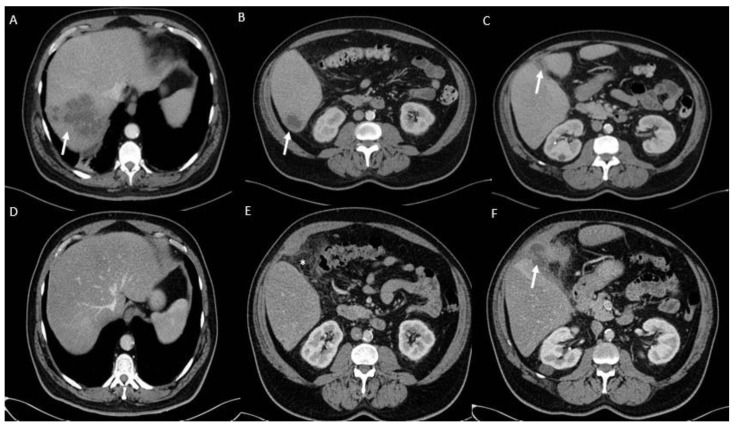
Abdominopelvic computed tomography scan after contrast in the venous phase. Several liver abscesses are seen as polylobulated hypodense lesions with peripheral enhancement in segments 7 (A) and segment 6 (B), while there is no abscess around the gallbladder bed (C). Resolution of the hepatic abscesses is seen in segments 7 (D) and segment 6 (E). However, a new abscess has developed in the gallbladder bed (arrow in (F)) with significant inflammatory changes in the surrounding mesocolonic fat, which were not present previously.
